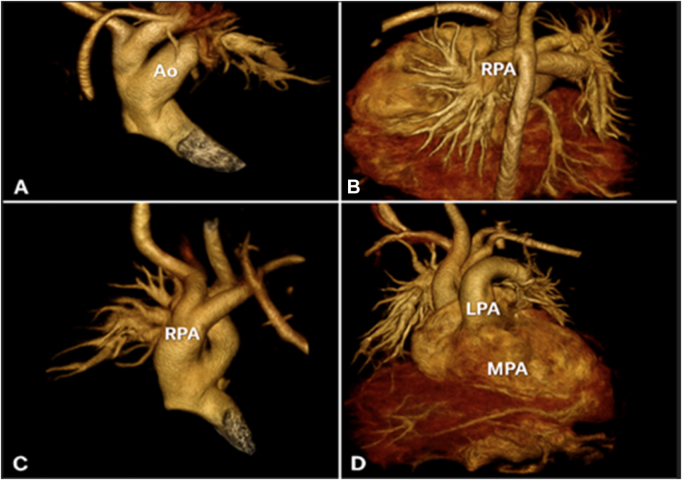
Preoperative 3D volume-rendered CT angiography demonstrating an anomalous origin of the right pulmonary artery (RPA) from the ascending aorta. (A, C) Oblique views showing the RPA arising separately from the aorta, coursing toward the right lung. (B, D) Additional views highlighting the spatial relationship between the aortic origin of the RPA and the normally arising left pulmonary artery (LPA) from the main pulmonary artery (MPA).
